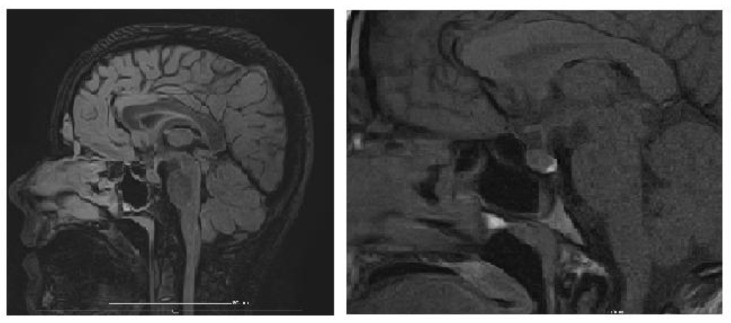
Brain MRI (dynamic post-contrast sequence) showing a 3 × 2 mm pituitary microadenoma.
